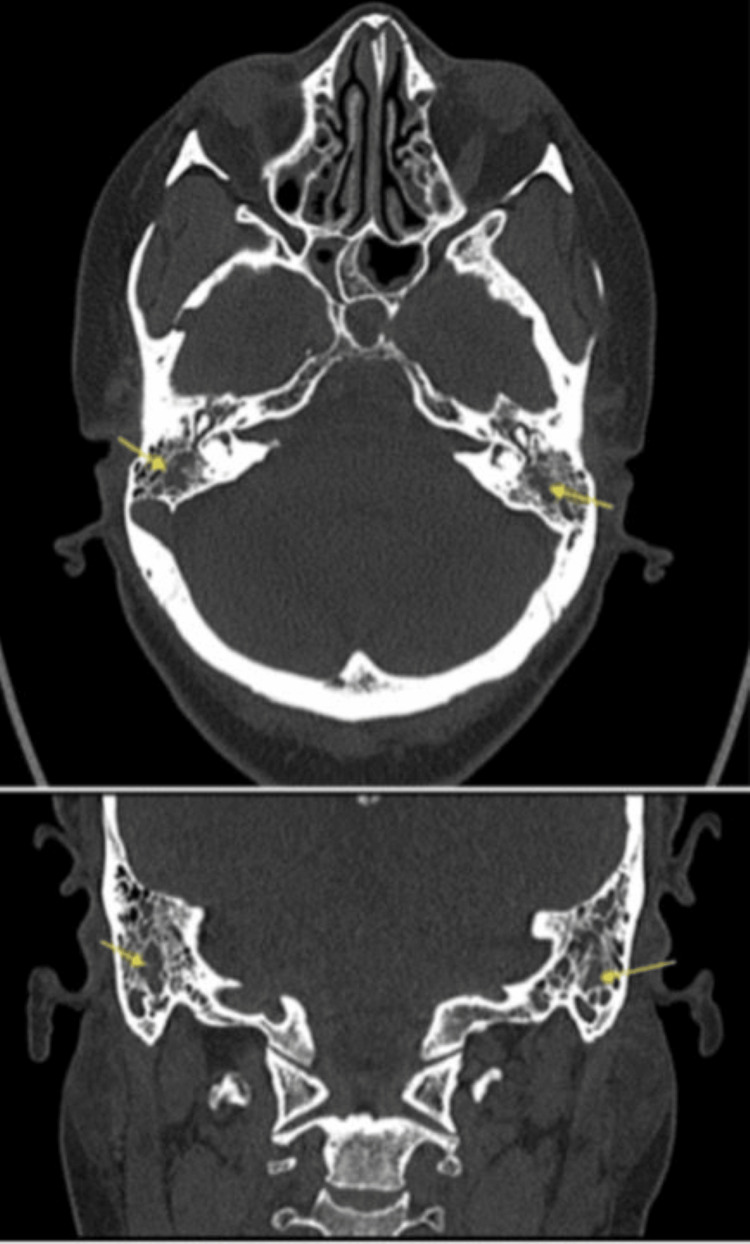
Bilateral mastoiditis The top image shows a cross-sectional view of CT Gead.  Arrows pointing at opacification (normally air) of bilateral mastoid air cells and no bony destruction, suggestive of bilateral mastoiditis. Similar changes are seen in the bottom image (arrows pointing at opacification in mastoid air cells).
